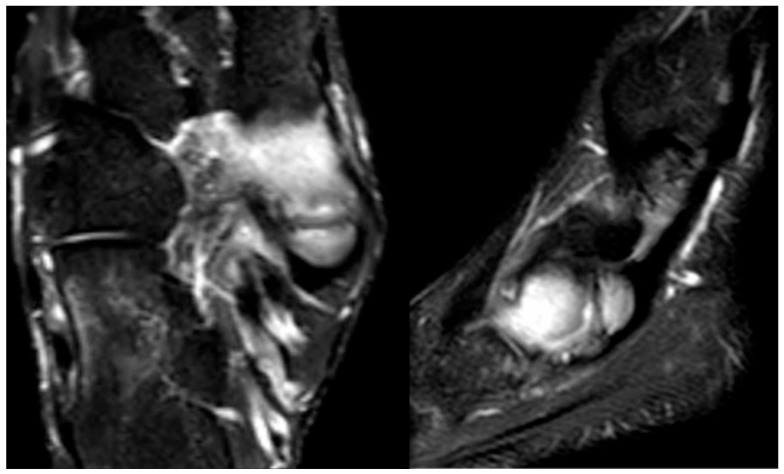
MRI of an 11-year-old symptomatic female patient showing bone marrow edema within the accessory navicular.
